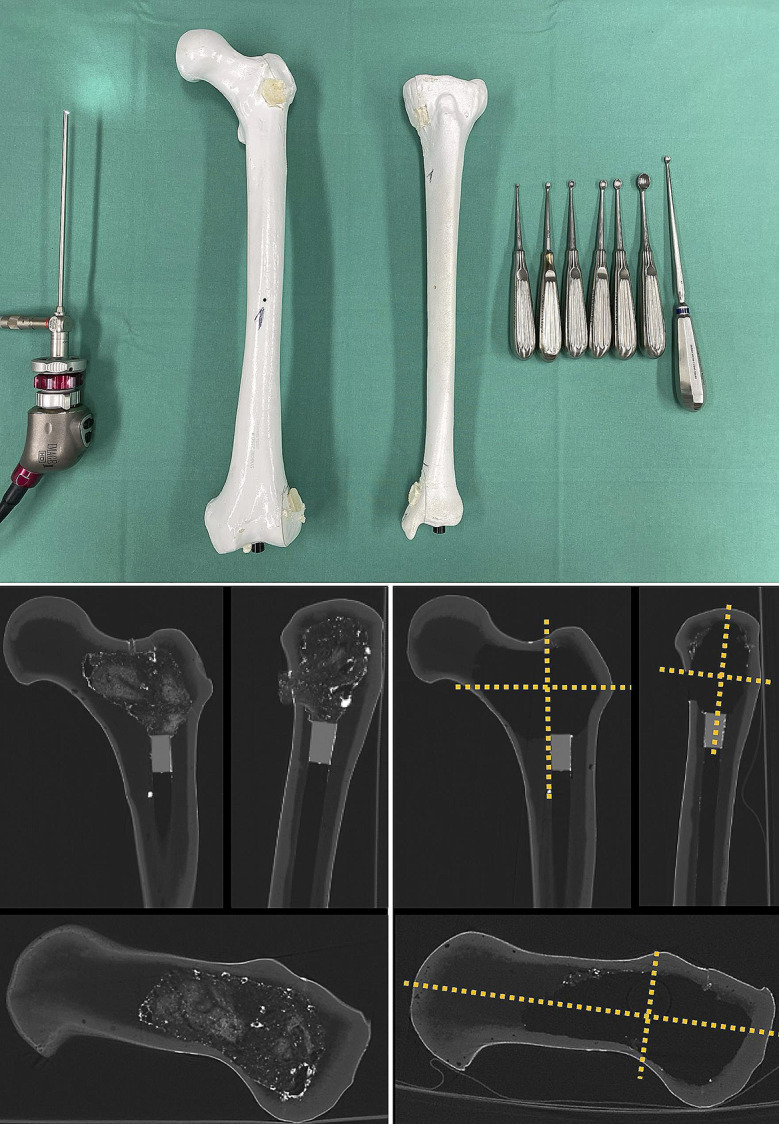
Experimental built-up. (Top) Picture of the experimental built-up showing endoscope (left), prepared femoral and tibial sawbone (middle) and curettes used (right). (Bottom, left) Pre-interventional CT scan of a proximal femoral sawbone in 3 planes (coronal, sagittal, axial) depicting the cement restrictor to seal the medullary canal and the contrast-enhanced foam used to mimic the lesion. (Bottom, right) CT-scan in 3 planes (coronal, sagittal, axial) of the same proximal femoral sawbone following curettage, with contrast-enhanced foam still visible at the lesion’s periphery. Orange lines define segments used for radiological assessment of RTT.
